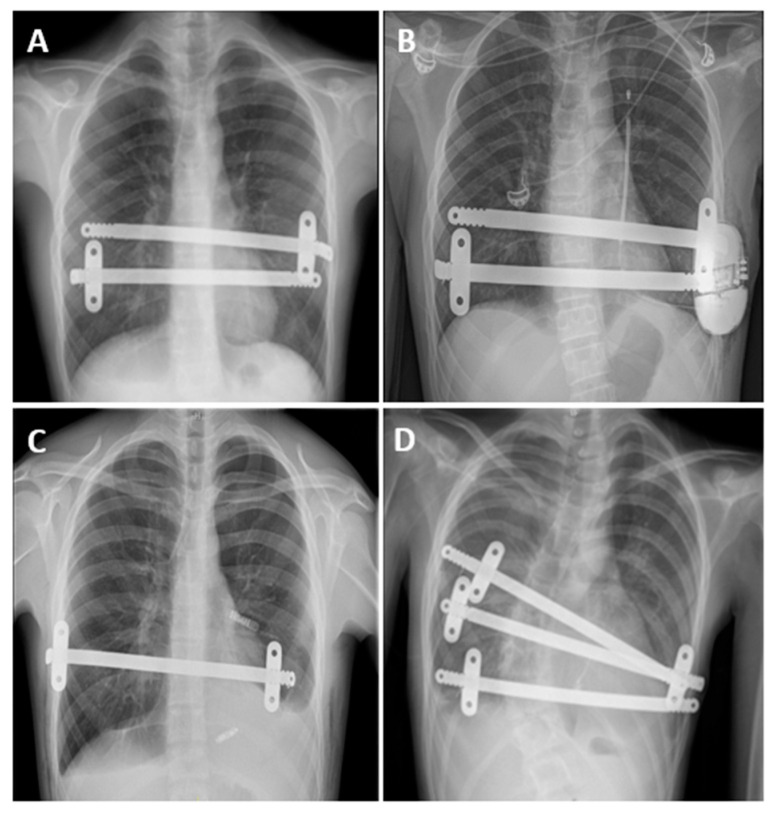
(A) Chest radiograph showing pectus repair with the insertion of two retrosternal metal bars and (B) after subcutaneous implantable cardioverter-defibrillator (S-ICD) implantation. (C) Chest radiograph showing pectus repair with the insertion of one retrosternal metal bar and MLP. (D) Chest radiograph showing pectus repair with the insertion of three retrosternal metal bars in a Marfan patient with a residual right-sided postoperative pneumothorax.
